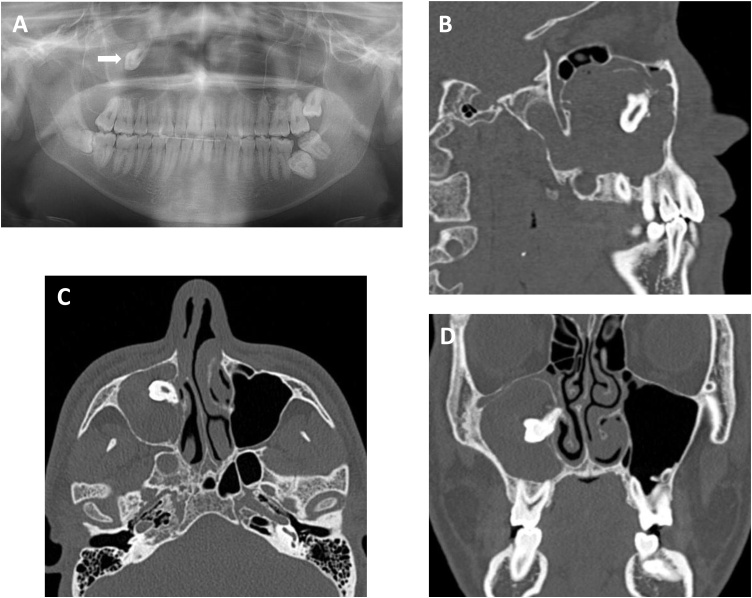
(A) Panoramic radiograph showing a dislocated third molar in the right maxillary sinus (arrow). (B) Sagittal CT scan shows OKC in the right maxillary sinus involving the third molar which has extended into the pterygoid process of the sphenoid bone near the skull base. (C) Axial CT scan. (D) Coronal CT scan.
